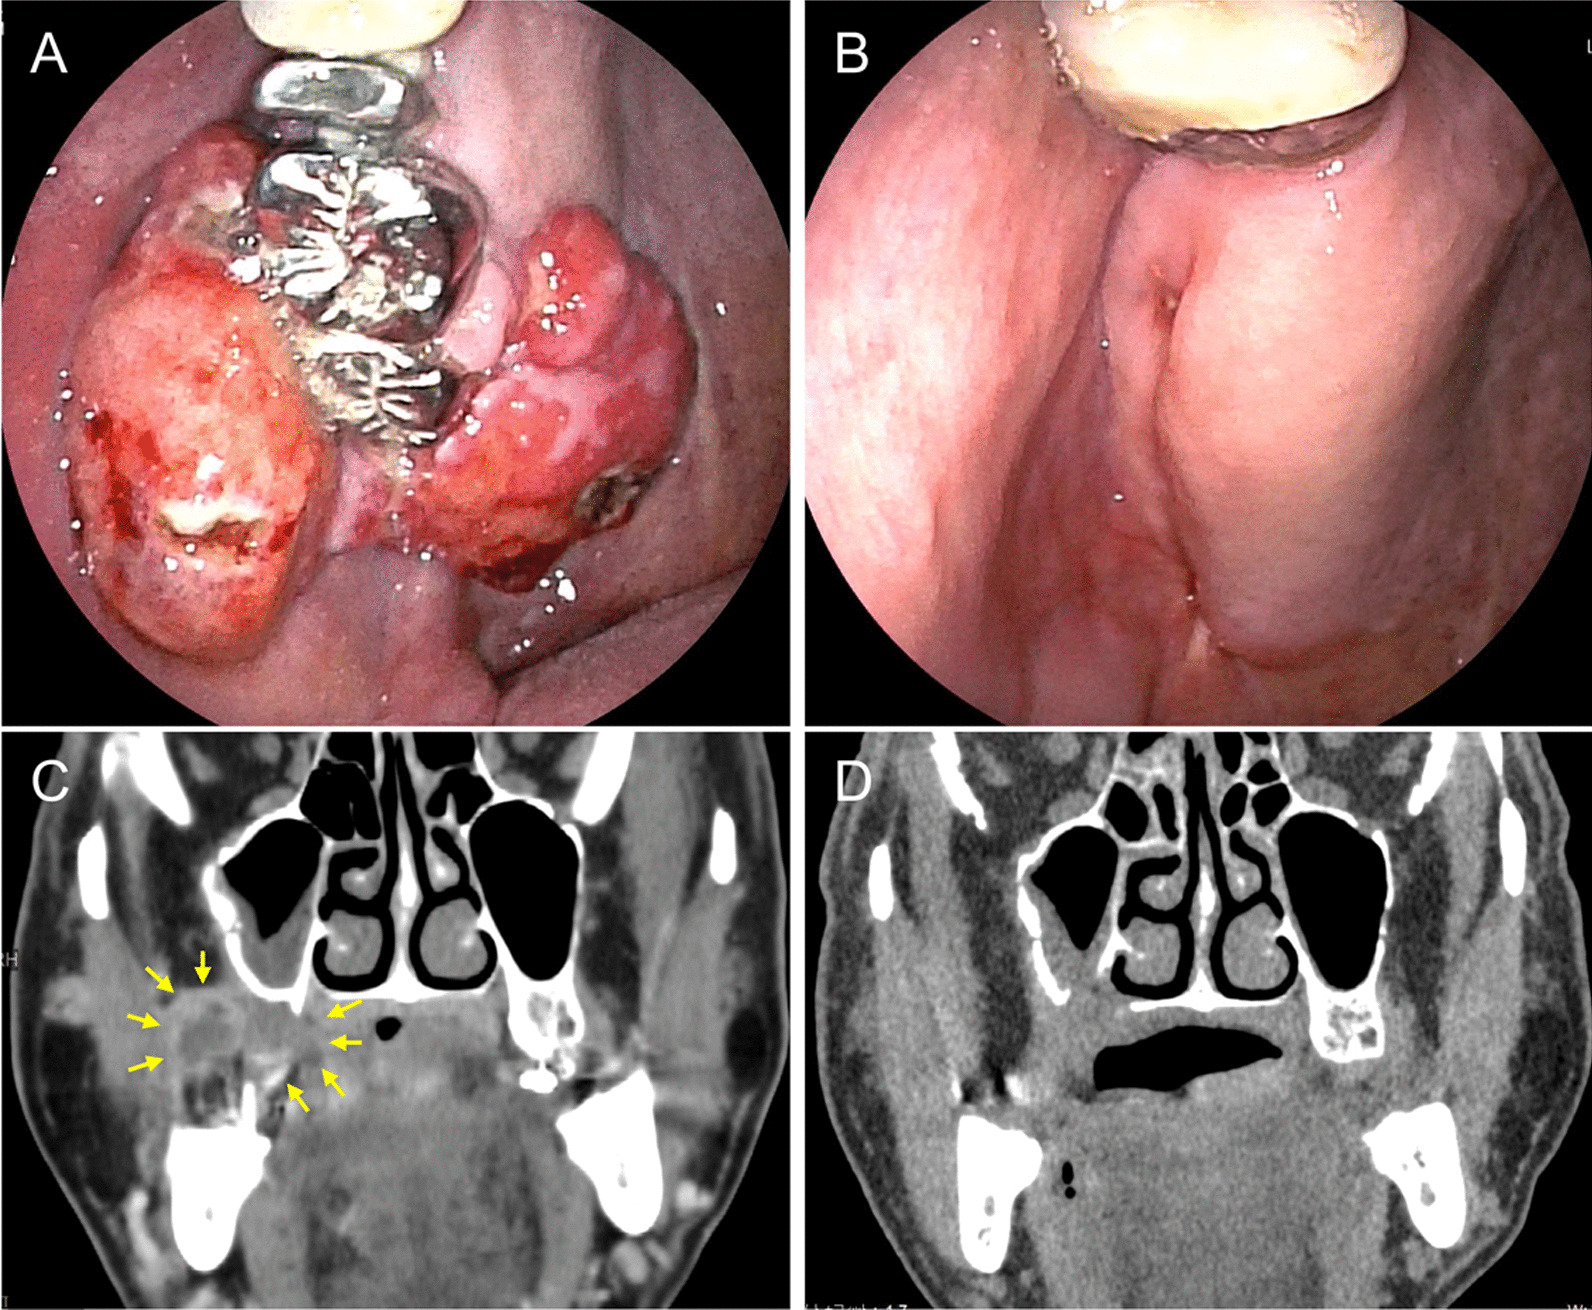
Comparison between pre- and postoperative findings. A and B Right maxillary gingival lesion site preoperatively (A) and 3 months postoperatively (B). C and D Coronal CT images preoperatively (C arrow: POF lesion) and 4 months postoperatively (D a fistula due to the bone defect of the maxillary sinus floor closed spontaneously)
